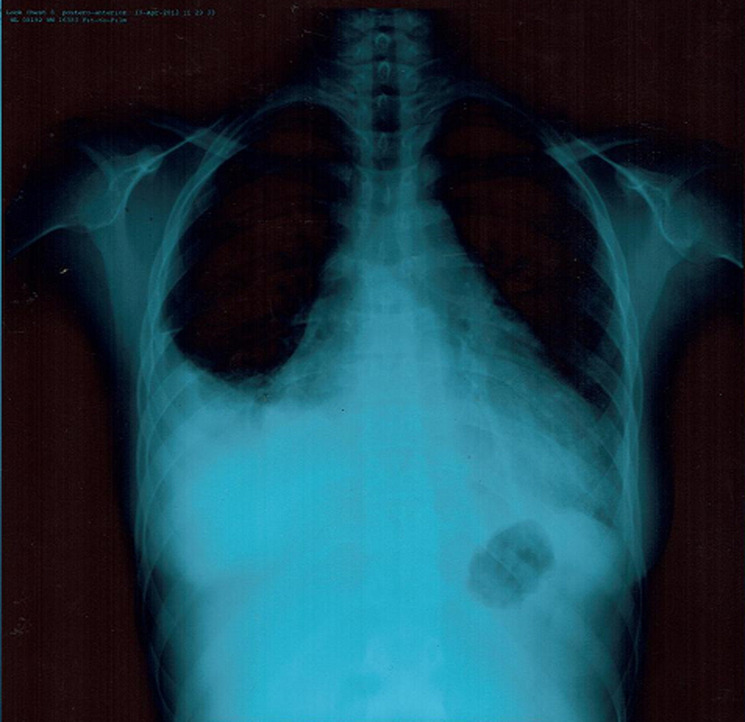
anteroposterior chest radiography showing enlargement of the cardiac silhouette with a right-sided pulmonary effusion
